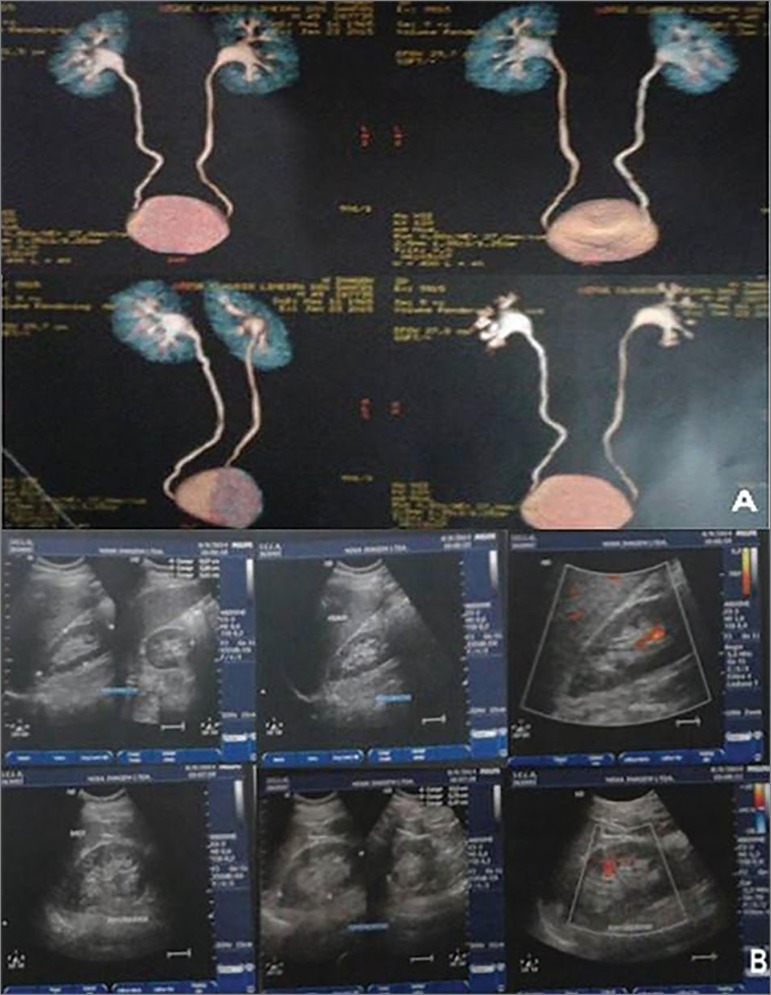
Abdominal CT (A) and Ultrasound (B) scan images showing mild renal pelvis ectasia and prostate calcifications.
